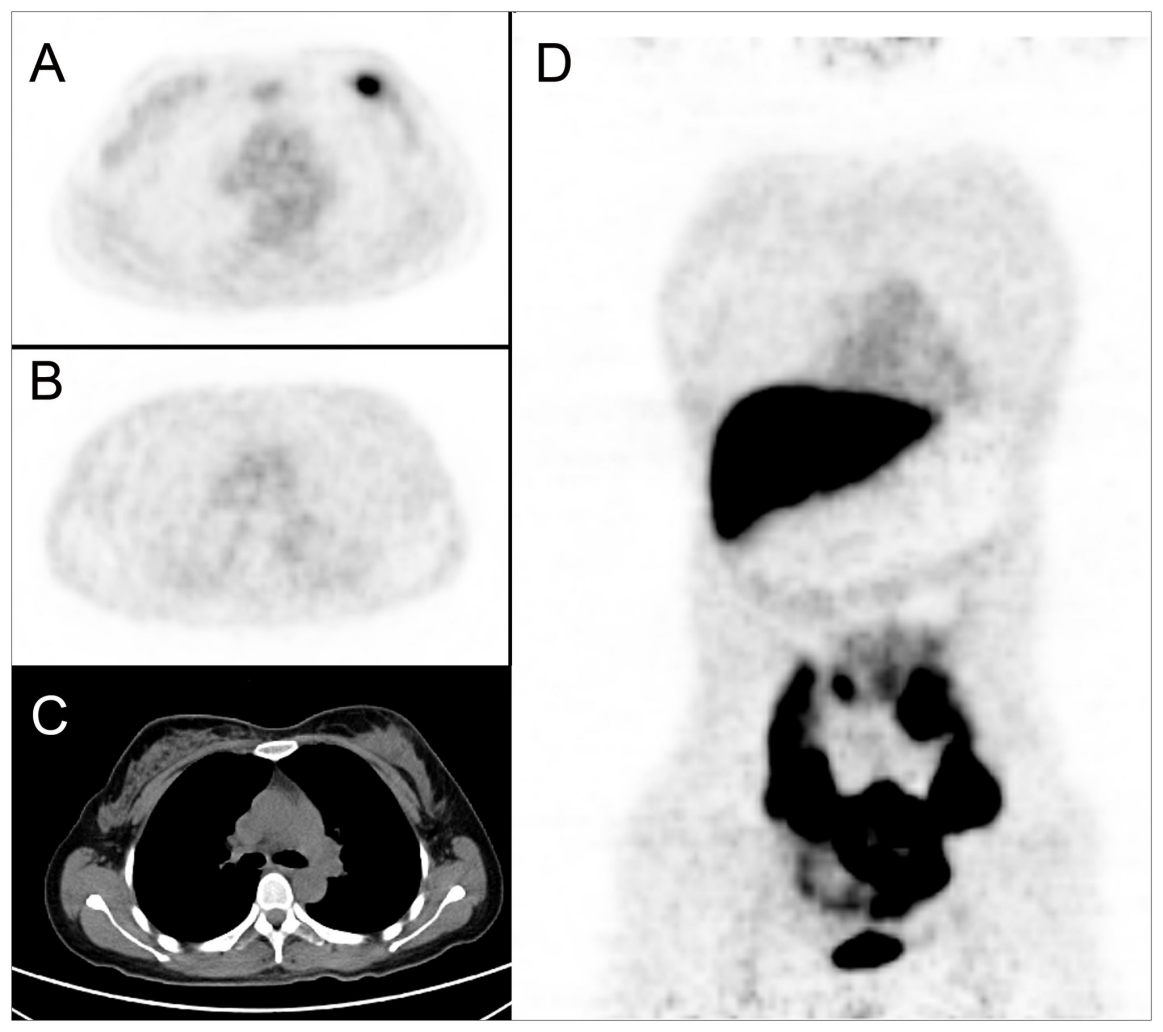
A 43-year-old female breast cancer patient scheduled to undergo adjuvant chemotherapy. We detected a mass in left breast (C. CT imaging), with a maximum diameter of 3.2cm. The tumor was FDG-avid with SUVmax of 8.99 (A. FDG PET/CT), but no obvious FES uptake was noted (B, D. FES PET/CT, SUVmax=1.1). After 4 cycles of NAC, the patient had mastectomy and the pathology showed it was grade A.
